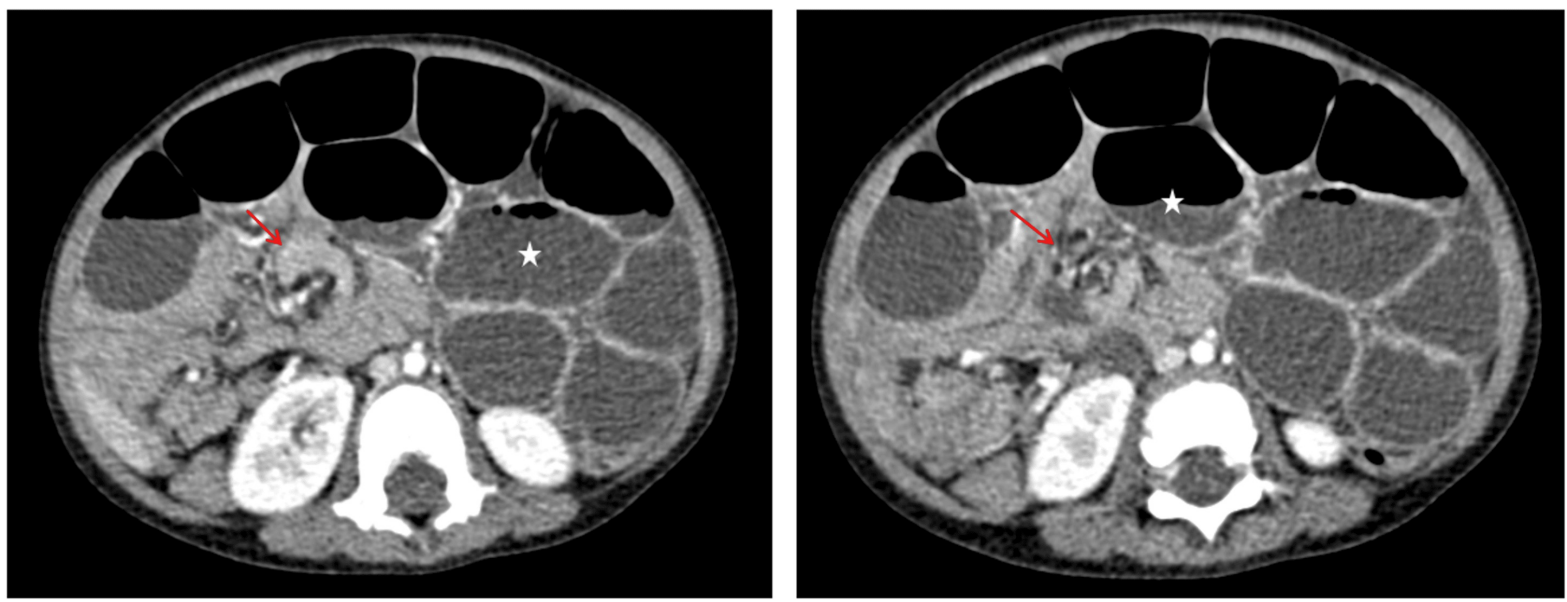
Axial slices of an abdominal CT scan showing small bowel obstruction (asterisk) upstream of a "whirlpool sign" (arrow), indicative of small bowel volvulus.
